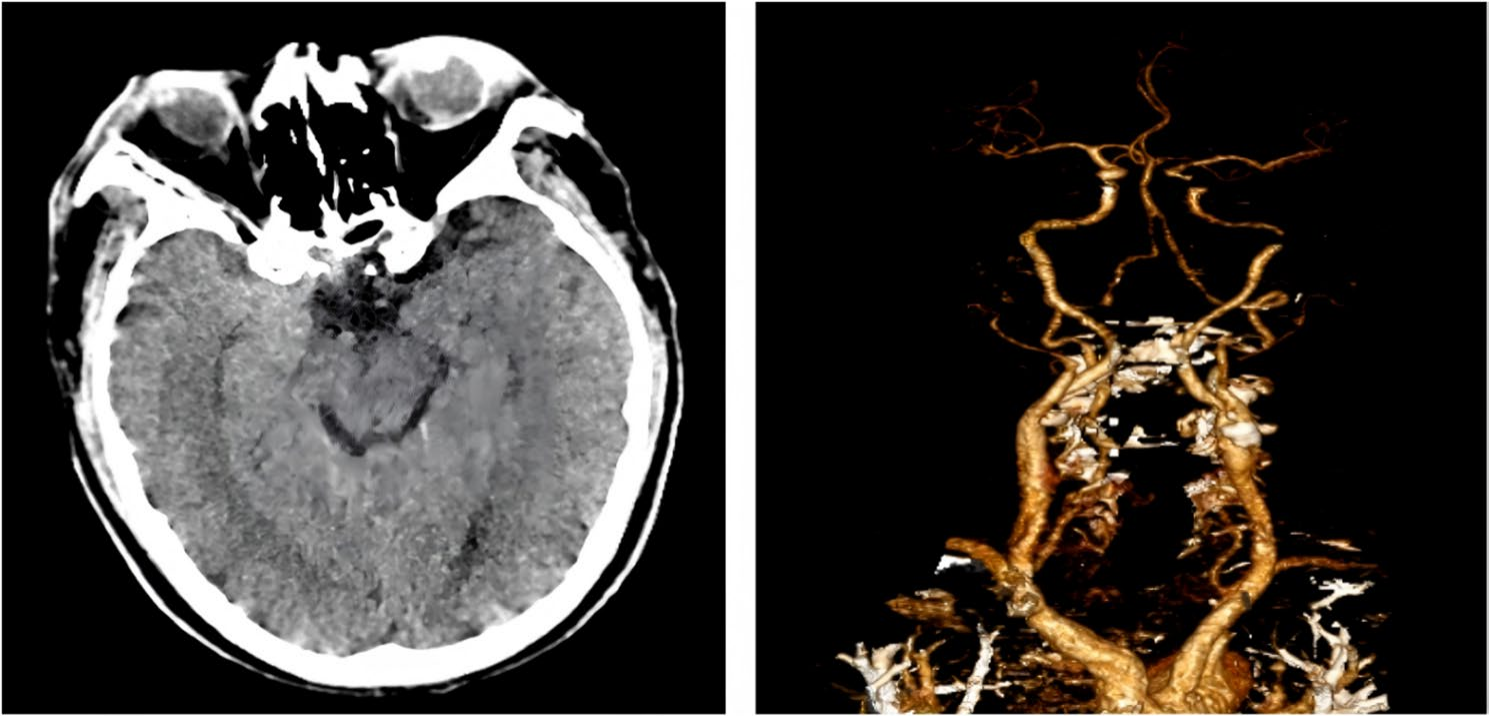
Brain CT Perfusion (CTP) Images No intracranial hemorrhage, infarction, or perfusion abnormalities
